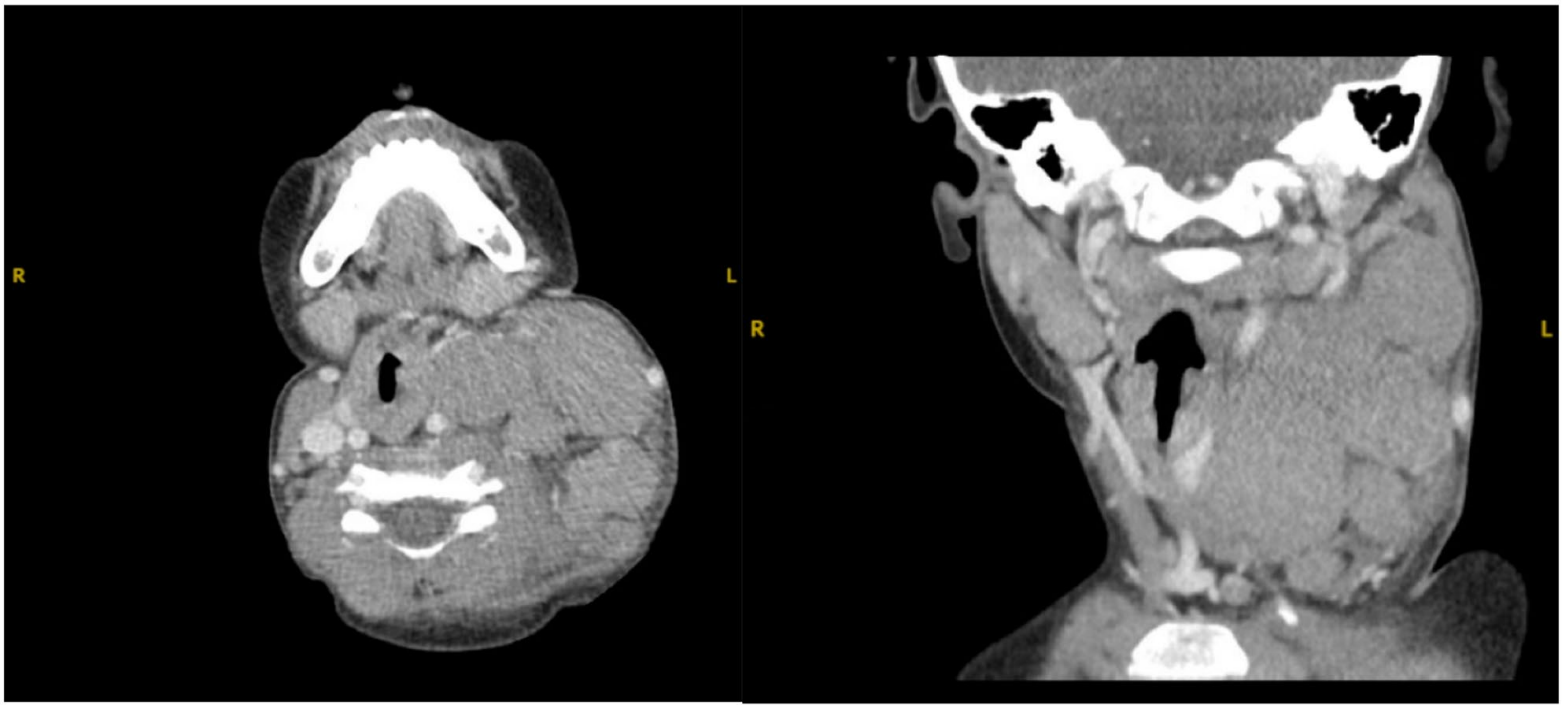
Computed Tomography Neck with Contrast: Axial and Coronal Views showing a large conglomerate of lymph nodes (11.5 cm) involving the left neck with significant mass effect
